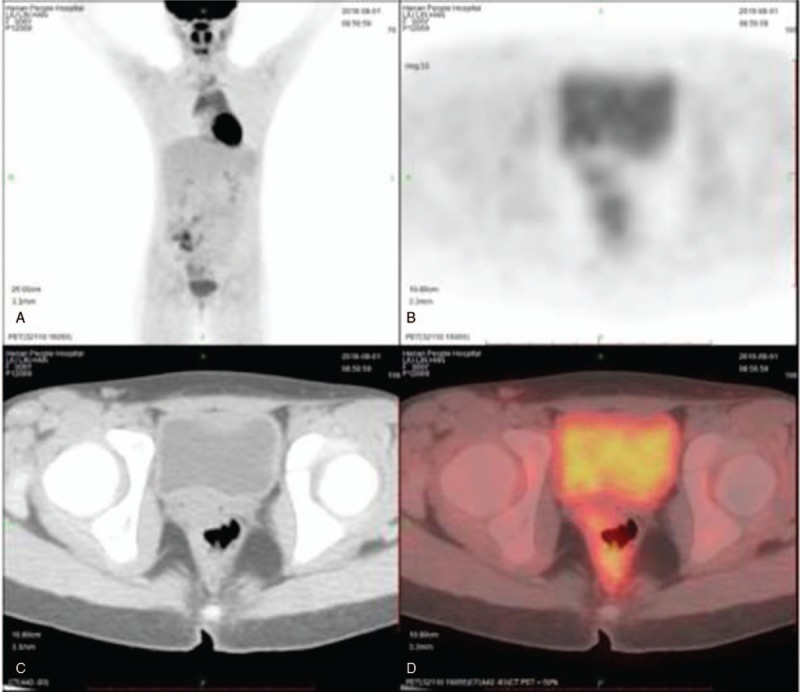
Positron emission tomography-computed tomography (PET-CT): preoperative (axial): the whole-body image scan with no other suspicious heterogeneously enhanced masses in the lymph nodes or any other organ. preoperative (coronal): a well-defined heterogeneously enhanced mass (metabolic map) at the tip of the right lateral wall of the vagina. preoperative (coronal): the lower abdomen and the cervix-vaginal area of the CT image. preoperative (coronal): The metabolic map of the same area as the Figure 2C photograph. PET-CT= positron emission tomography-computed tomography.
